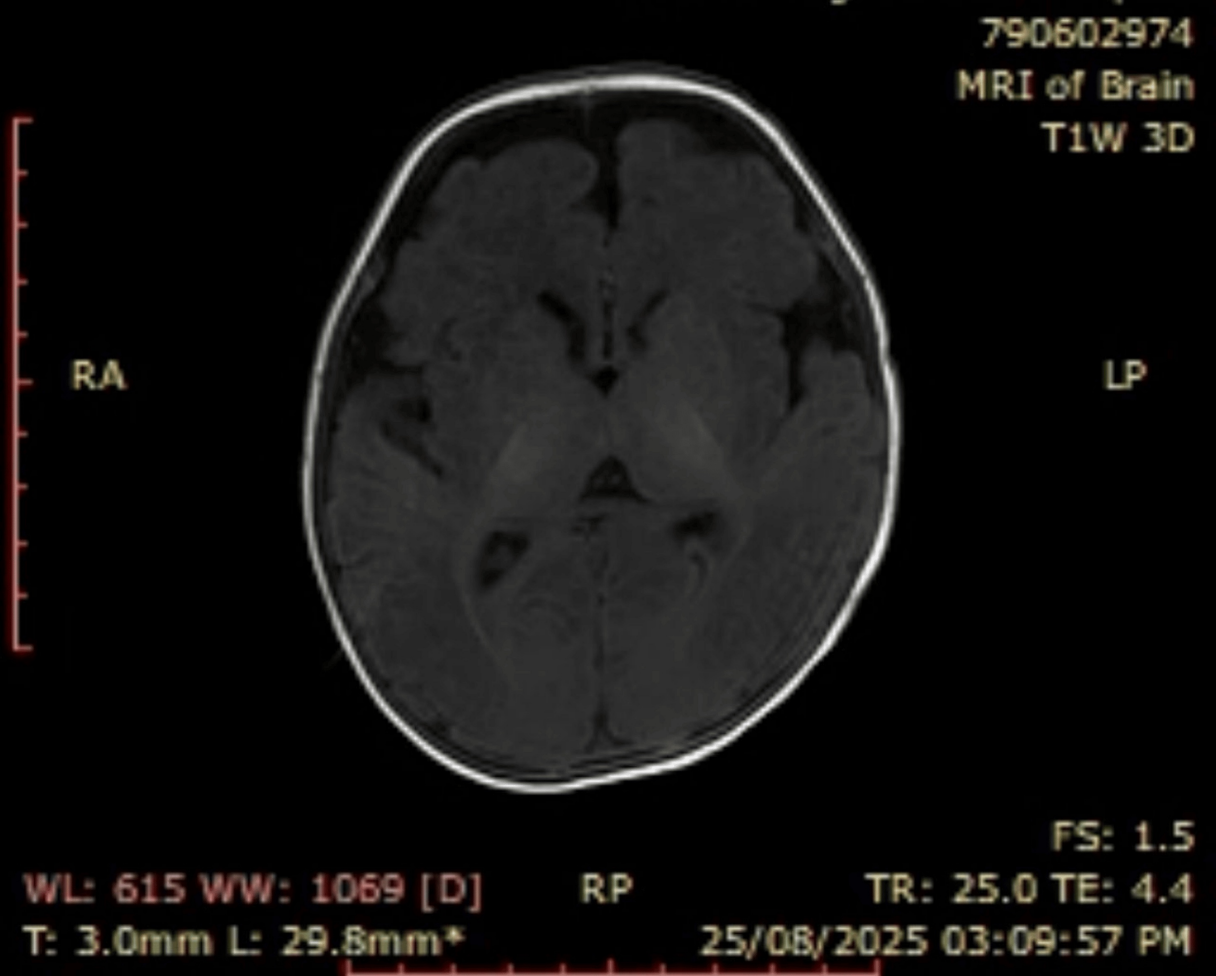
Axial T1-weighted MRI image of the brain, demonstrating normal parenchyma and myelination for age, with no structural abnormalities. MRI: magnetic resonance imaging
